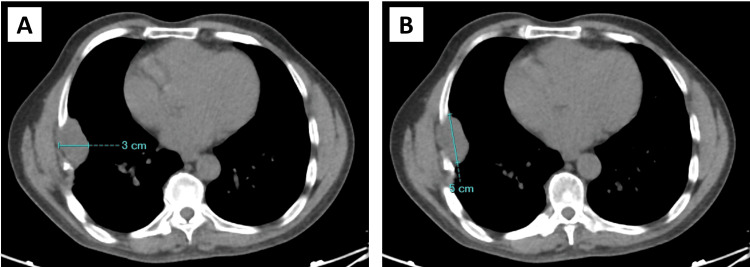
Measurement of the schwannoma based on low-dose CT scan CT: Computed tomography; pre-operative measurement using CT imaging estimated the size of the recurring schwannoma to be 3 cm in width (2A) and 5 cm in length (2B)
